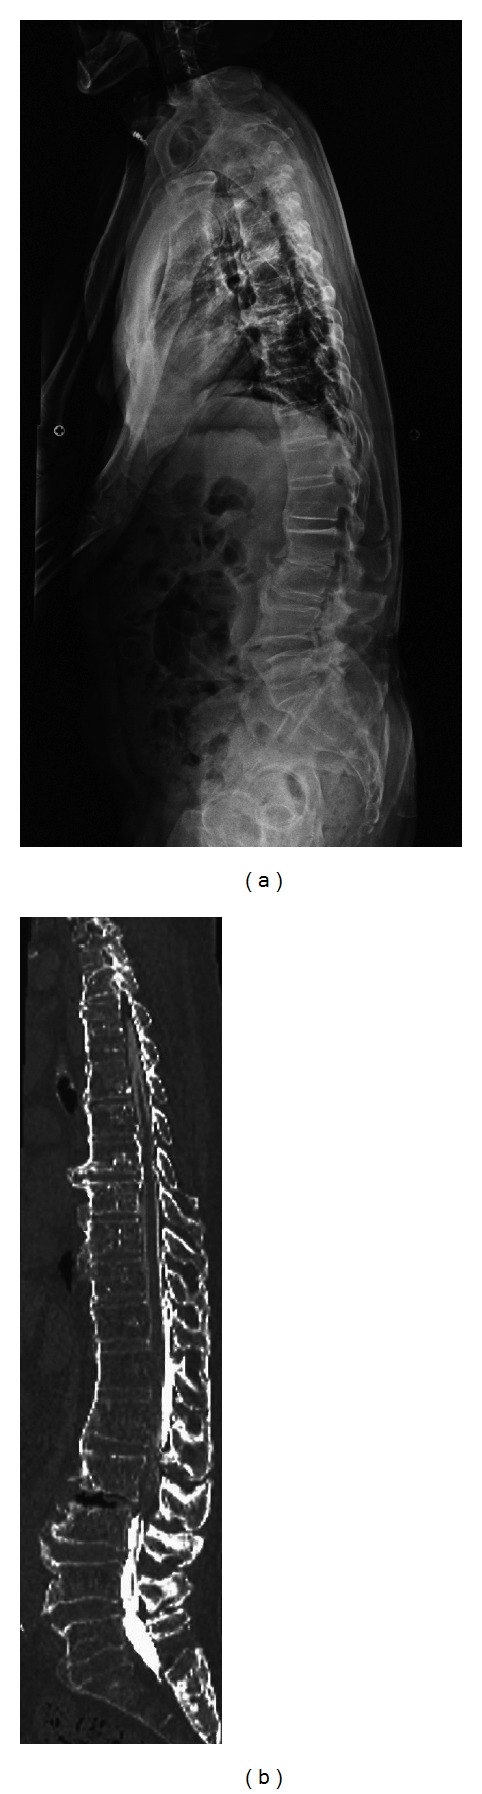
(a) Length in the standing position and lateral image in the standing position. (b) CT-MPR of the total length of the spine. The lateral X-ray image in the standing position showed no L2-L3 intervertebral space, but CT-multiplanar reconstruction images in the dorsal position showed a clearly opened L2-L3 intervertebral space.
